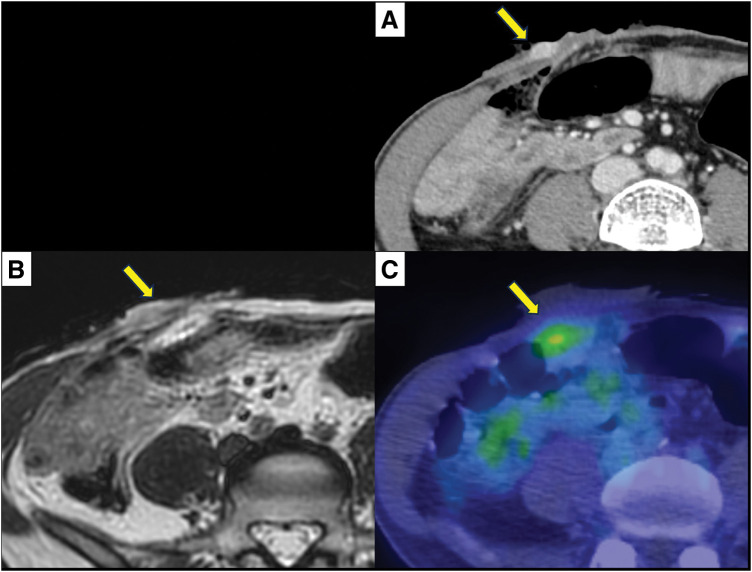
(A) Abdominal CT showed irregular, mild thickening of the peristomal skin surface with partial contrast enhancement (arrow). (B) Abdominal MRI revealed wall thickening with diffusion restriction at the stoma site without apparent infiltration into the rectus abdominis muscle (arrow). (C) FDG-PET/CT demonstrated an accumulation with an SUVmax of 3.0 in the intestinal tract near the stoma site, with no evidence of lymph node or distant metastasis (arrow). SUVmax, maximum standardized uptake value
